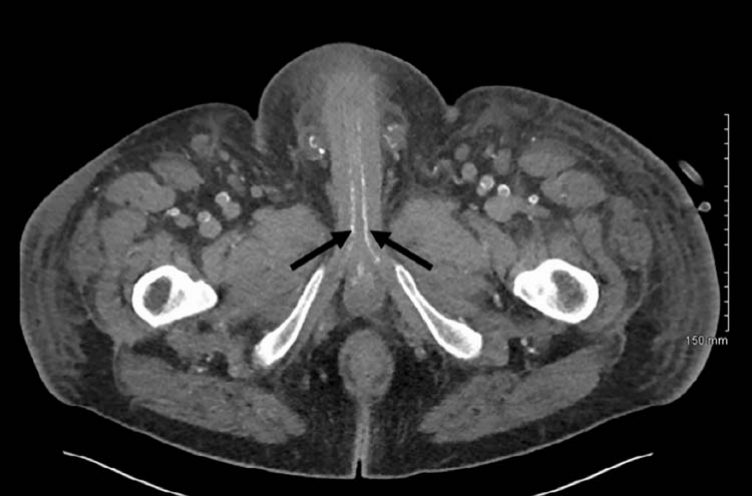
Axial slice of a non-contrast CT AP with arrows delineating calcified cavernosal arteries.
